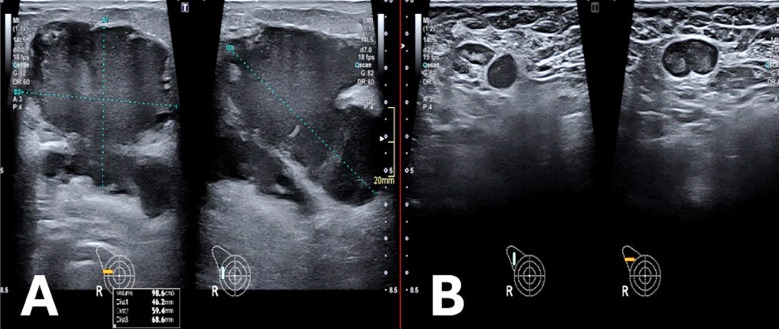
Ultrasound images of a young lactating female with breast inflammation showing. (A) fluid collection with internal echoes in right breast, consistent with lactational abscess - (B) axillary lymph node showing diffuse cortical thickening in accordance with reactive ipsilateral lymphadenopathy.
